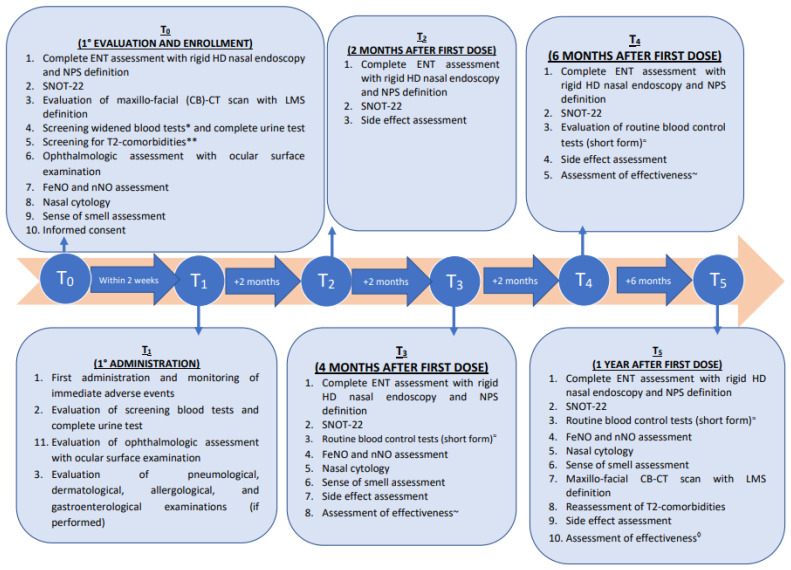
Flow-chart describing the diagnostic and therapeutic program scheduled for patients with severe chronic rhinosinusitis with nasal polyps (CRSwNP) and who are candidates for dupilumab. *Abbreviations: ENT: ear, nose, and throat; NPS: Nasal Polyp Score; LMS: Lund–Mackay score; SNOT-22: SinoNasal Outcome Test score; CB-CT: cone-beam computed tomography; FeNO: fraction of exhaled nitric oxide; nNO: nasal nitric oxide.* *: including complete blood count and differential, lymphocyte subpopulation analysis, eosinophils count; complete liver enzymes; serum protein; serum creatinine; serum reactive C-protein; serum IgA, IgG, IgM, IgE level; serum peri-nuclear antineutrophil cytoplasmatic antibodies, extractable nuclear antigens; creatine phosphokinase serum level, eosinophil cationic protein serum level; : in case of suspected or known T2-related comorbidities address the patients to further (dermatological, allergological, pneumological, and gastroenterological) assessments; ~: based on documented improvement in NPS and/or SNOT-22 and/or sense of smell assessment; ≈: including complete blood count and differential, eosinophils count; serum IgE level; eosinophil cationic protein serum level; ◊: based on documented improvement in NPS and/or SNOT-22 and/or sense of smell assessment and/or LMS and/or comorbidities.
